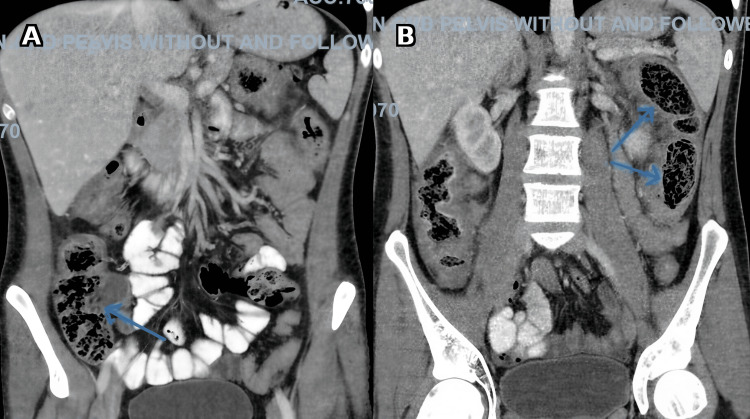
Contrast-enhanced CT of the abdomen Coronal images show luminal narrowing at the ascending colon (blue arrow) (A) and at the splenic flexure and descending colon (blue arrows) (B).
